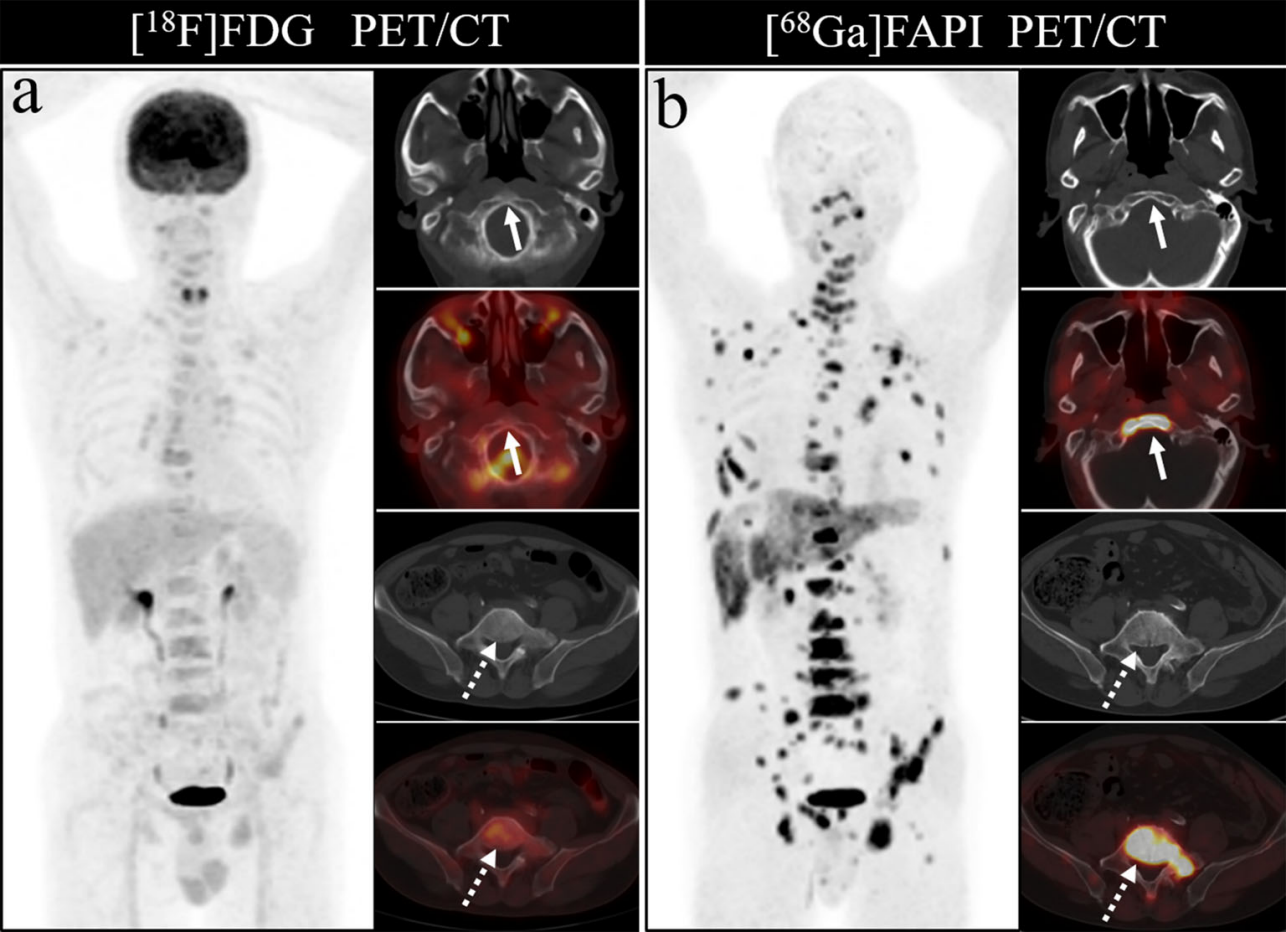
A 63-year-old man was received [^18^F]FDG and [^68^Ga]Ga-DOTA-FAPI-04 PET/CT (A, B) to evaluate the new diagnosed lung cancer. The MIP image (A, B) of [^18^F]FDG and [^68^Ga]Ga-DOTA-FAPI-04 PET/CT demonstrated multiple bone lesions. On the selected axial images, [^18^F]FDG PET/CT (A) only showed slight [^18^F]FDG activities in the clivus (arrows) and lumbar vertebral (dashed arrows). On the contrary, intense FAPI uptakes in the clivus (arrows) and lumbar vertebral (dashed arrows) were obviously observed on [^68^Ga]Ga-DOTA-FAPI-04 PET/CT.
